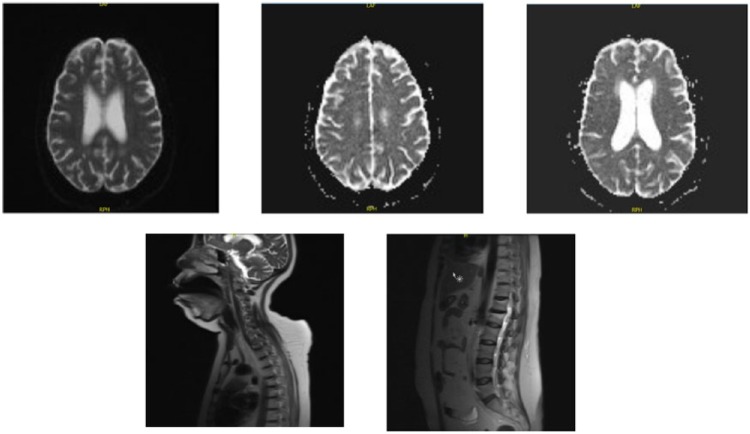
MRI at diagnosis. MRI Brain: diffuse extensive leptomeningeal enhancement with components of nodularity. Additional punctate foci of enhancement involve the brain parenchyma. Some associated high T2 and FLAIR signal at corresponding locations. Additional patchy areas of high T2 and FLAIR signal involving periventricular white matter, particularly about the frontal horns. MRI Cervical Cord: Subtle punctate nodular foci of enhancement along the cervical cord surface, patchy high T2 signal involves the upper cervical cord extending to the C2 level. MRI Thoracic Cord: Small nodular punctate foci of enhancement along the thoracic cord surface.
